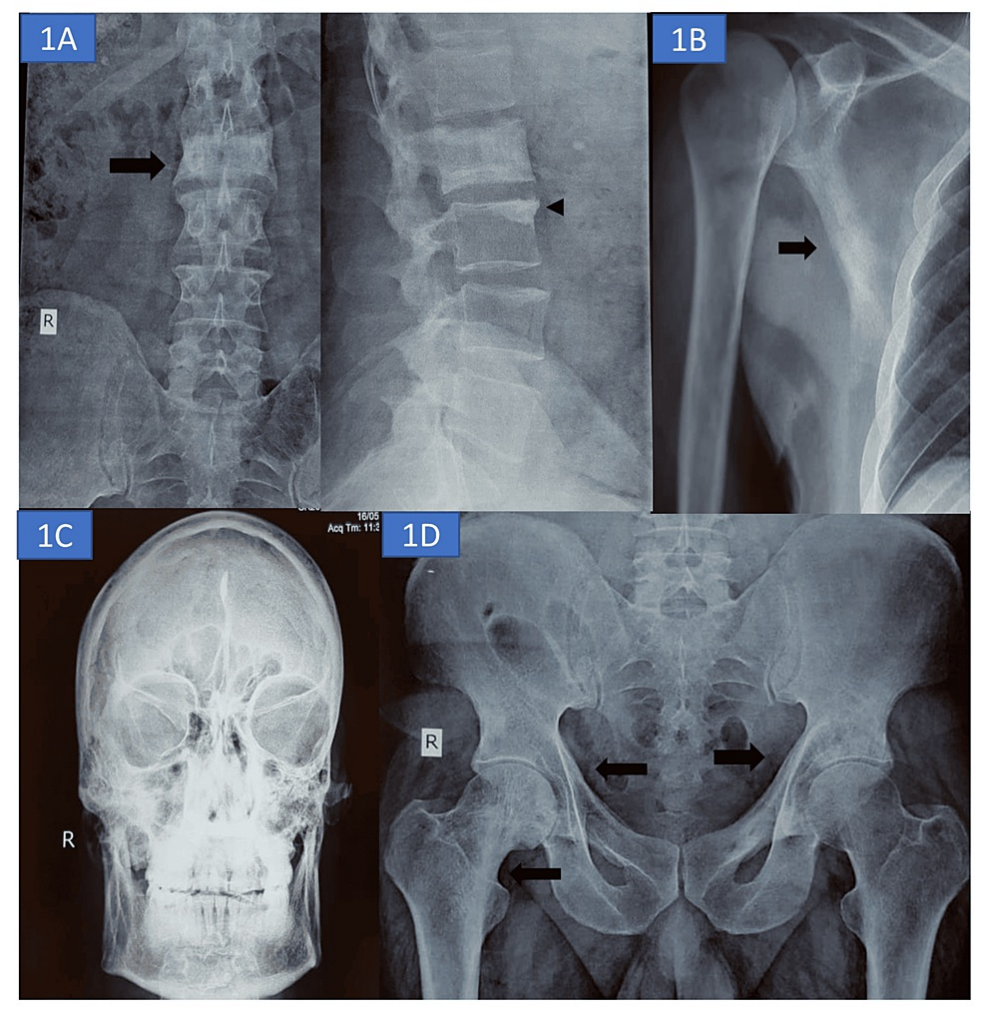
Skeletal radiograph (A) X-ray of the lumbar spine AP and lateral view shows diffuse sclerosis of the L2 vertebral body (ivory vertebra) with coarsened trabeculae (arrow). Degenerative sclerosis of the L3 upper end plate is also seen (arrowhead). (B) Cortical thickening of the lateral scapular margin is seen in the right shoulder. (C) X-ray of the skull AP view shows diffuse widening, thickening, and sclerosis of the diploic spaces of the skull bones. (D) X-ray of the pelvis AP view shows thickened bilateral iliopectineal line and coarsened trabeculae of the inferomedial right femoral neck (black arrows).
